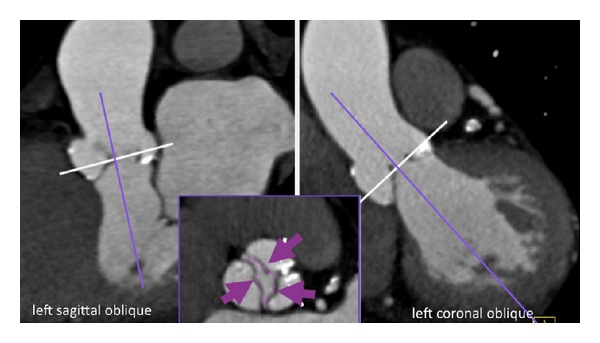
Aortic stenosis: Aortic valve area (AVA) sizing by CT*. Using 3-multiplanar reformations (MPR), from left sagittal oblique (left) and left coronal oblique (right) views, a cross-sectional view of the aortic valve is generated (*lower mid image*). The white line indicates the plane of the cross-sectional axial oblique view in the low midimage inlay, which allows quantification of the inner aortic valve orifice area (AVA) (pink arrows). This area is traced by a digital caliper and reflects a marker for the severity of aortic stenosis (<1 cm^2^: severe critical). Valve morphology was bicuspid (fused raphe).
